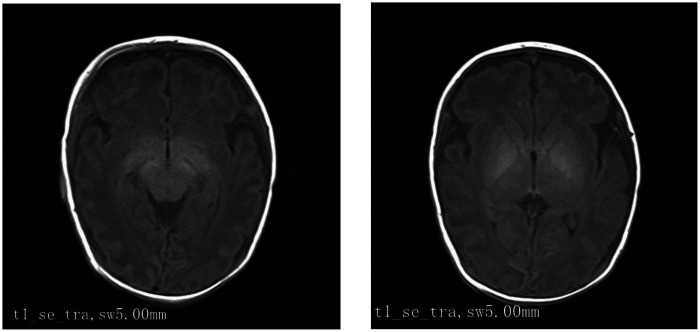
Cranial MRI revealed hyperintensity of bilateral globus pallidus on T1WI.
